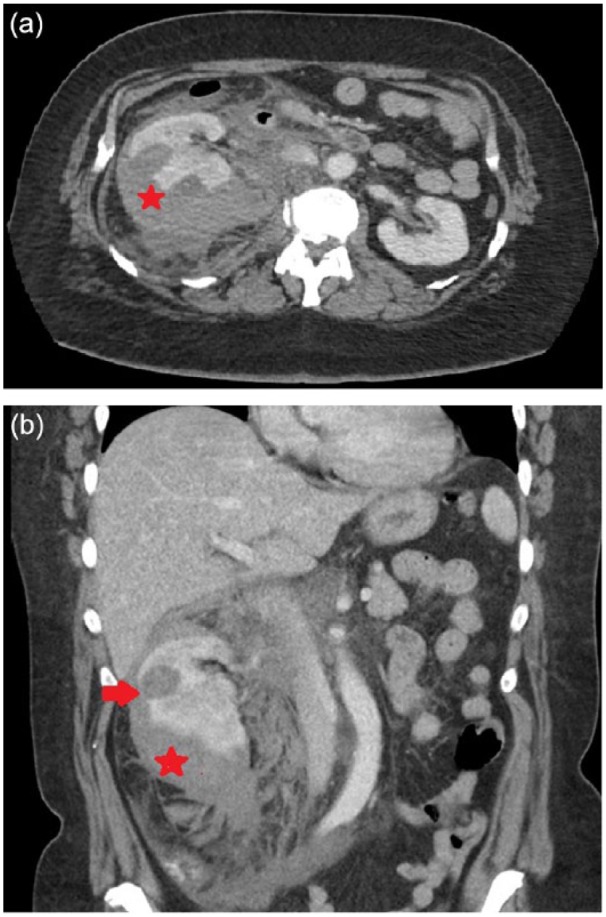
(a) Axial and (b) coronal contrast-enhanced computed tomography scan of the abdomen showing a right renal extra-capsular hematoma.
